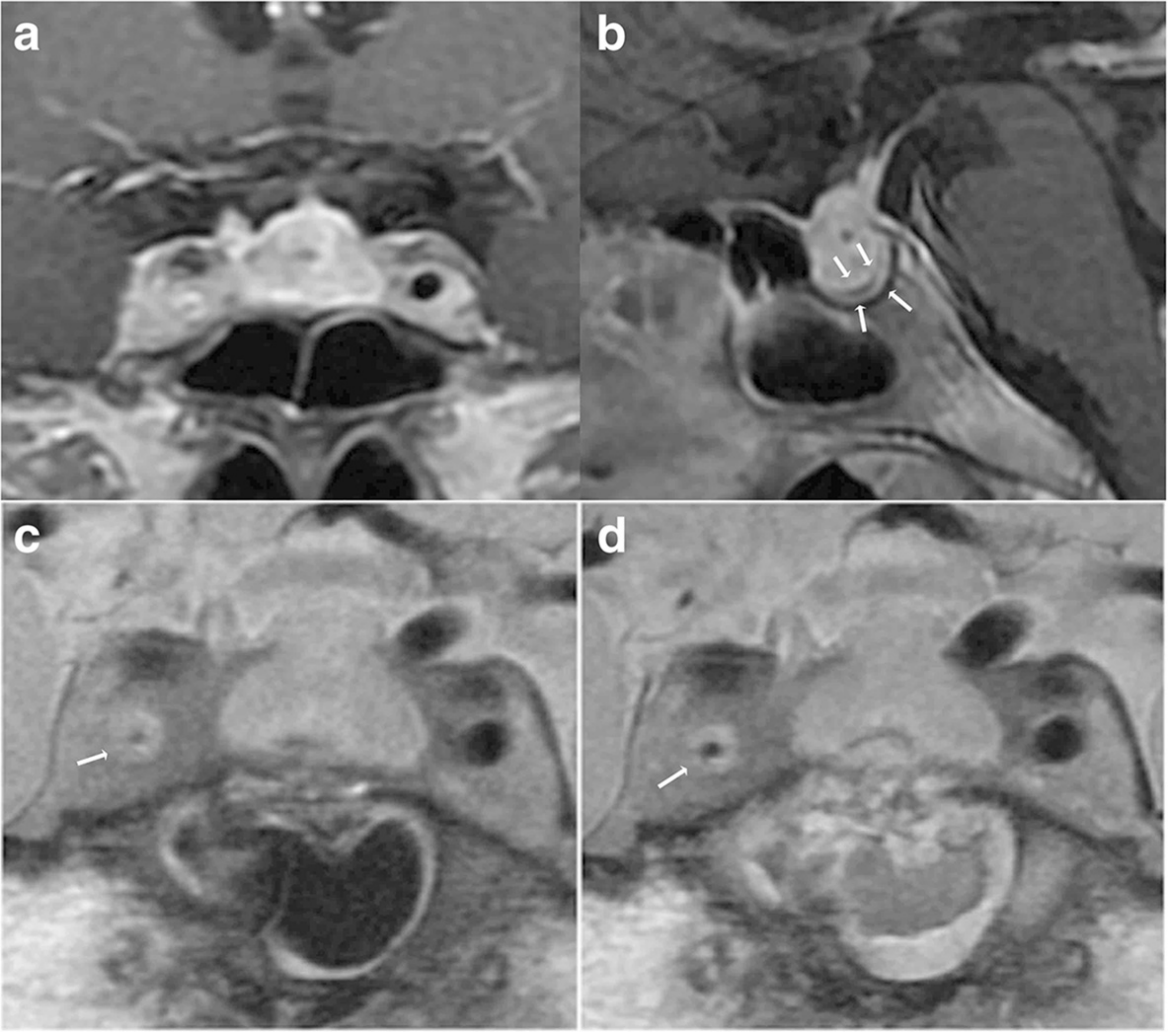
Chronological MR images of Case 2. (a, b) Preoperative sagittal T1-weighted MR images with gadolinium revealing a sellar lesion extending to the suprasellar cistern (a), and markedly thickened dura mater (b). (c) Preoperative coronal plaque MR image revealing the thickened wall of the right internal carotid artery and the severely narrowed lumen. (d) Postoperative coronal plaque MR image showing improved right internal carotid artery stenosis.
